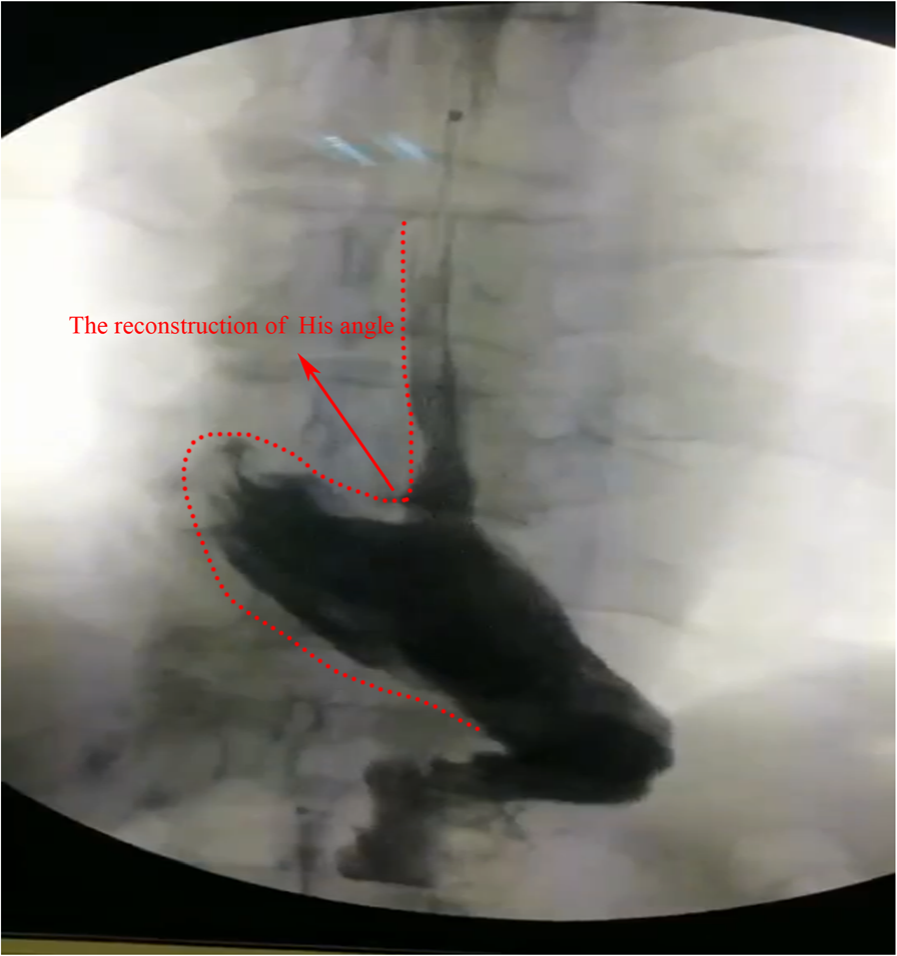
Reconstruction of the gastric fundus and His angle on upper gastrointestinal radiography
